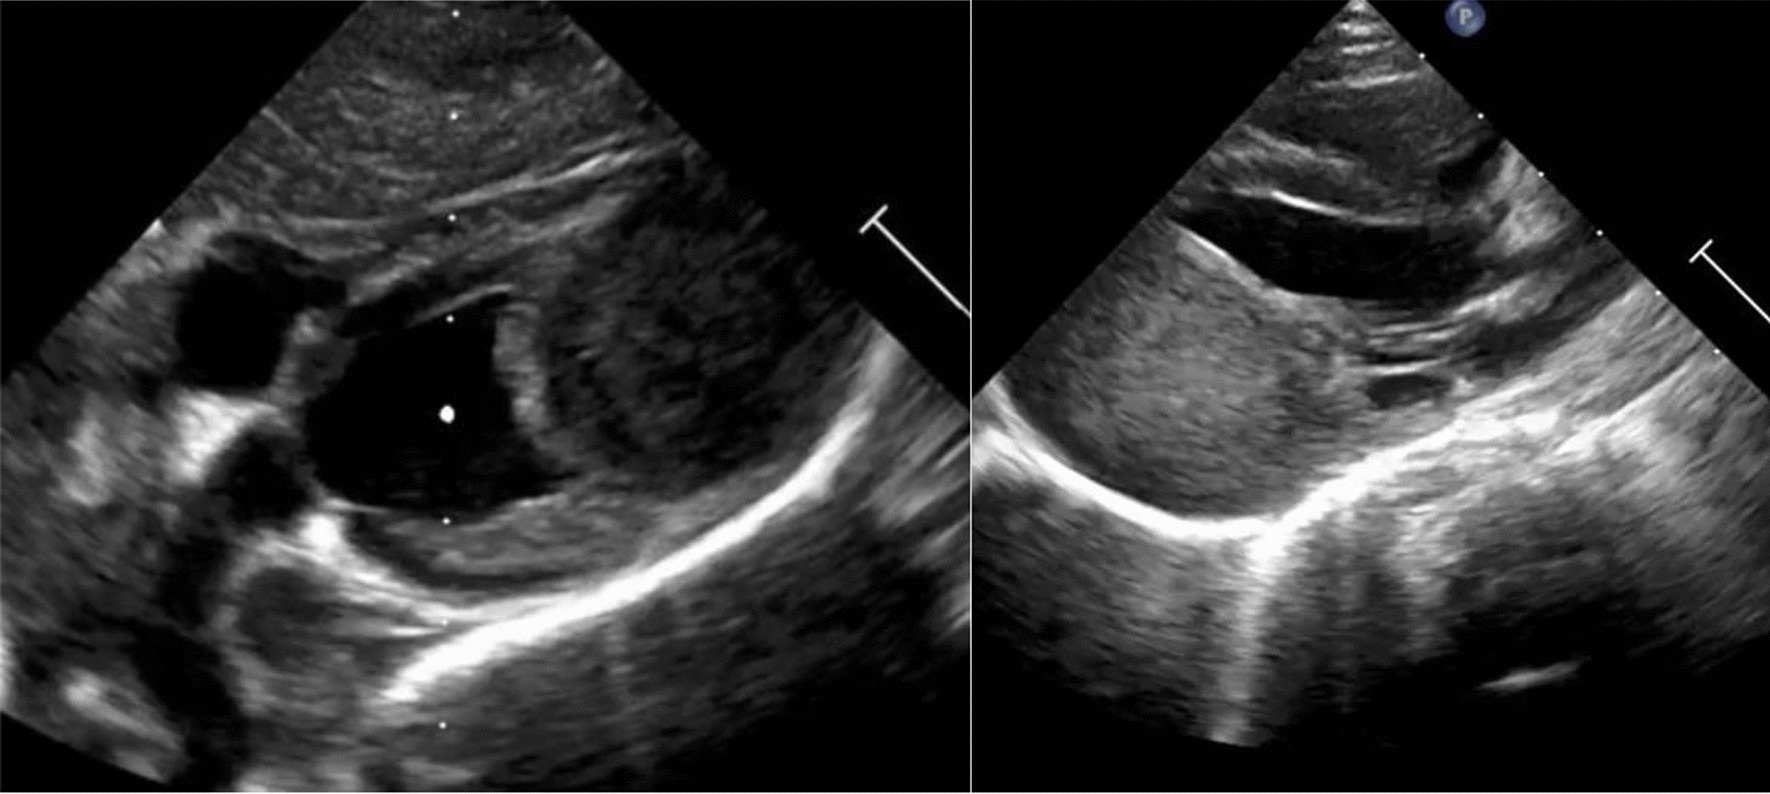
echocardiogram girl. Legend: Two-dimensional echocardiogram showing the tumor arising from the left ventricular wall (modified subcostal view, left) and infiltration of the tumor into the papillary muscle of the mitral valve (parasternal long axis, right)
